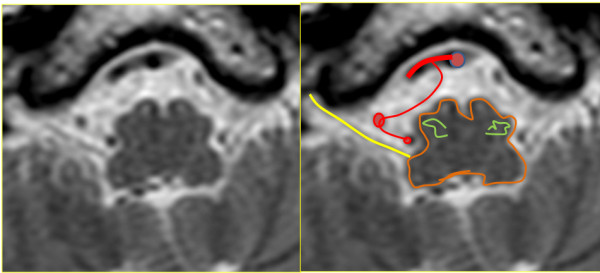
Magnetic resonance imaging scans: high-resolution, T2-weighted, transverse plane. Vascular contact between the glossopharyngeal nerve (yellow) exiting the retro-olivary fossa (olive green) and right posterior inferior cerebellar artery (PICA).
